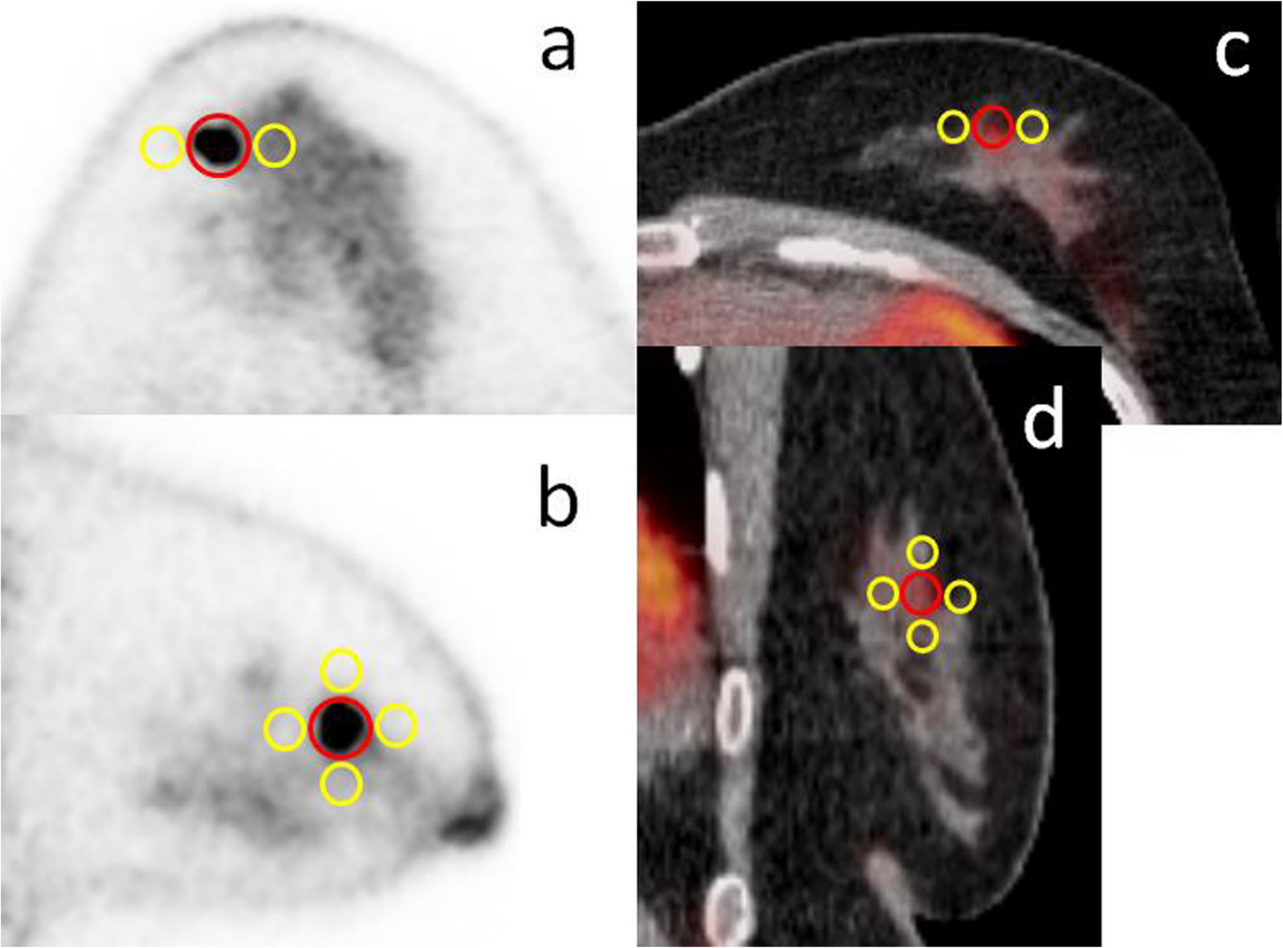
Positioning of the volume of interest (VOI) for the measurement of clinical PET images. A spherical VOI (red) on FDG uptake of the tumour and 5 (or 6) spherical VOIs (yellow) were placed to calculate the TBR. Transaxial and sagittal images of dbPET (a, b) and whole-body PET/CT (c, d)
